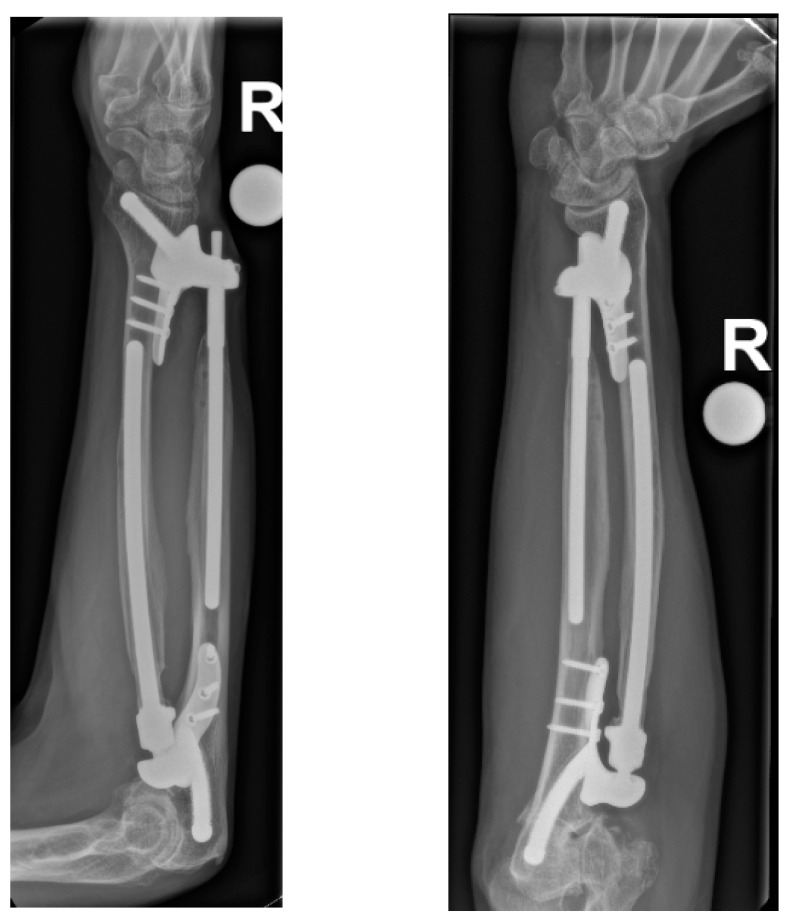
Follow-up X-ray at 2.5 years after combined proximal and distal DRUJ replacement.
